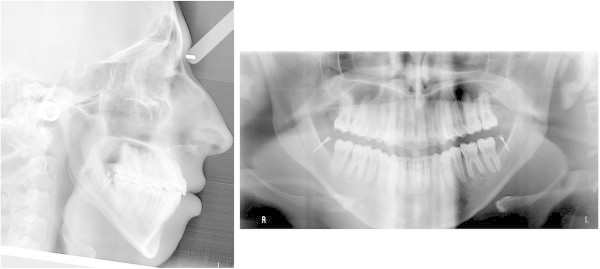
Posttreatment cephalogram and panoramic radiograph with miniscrew inserted.
